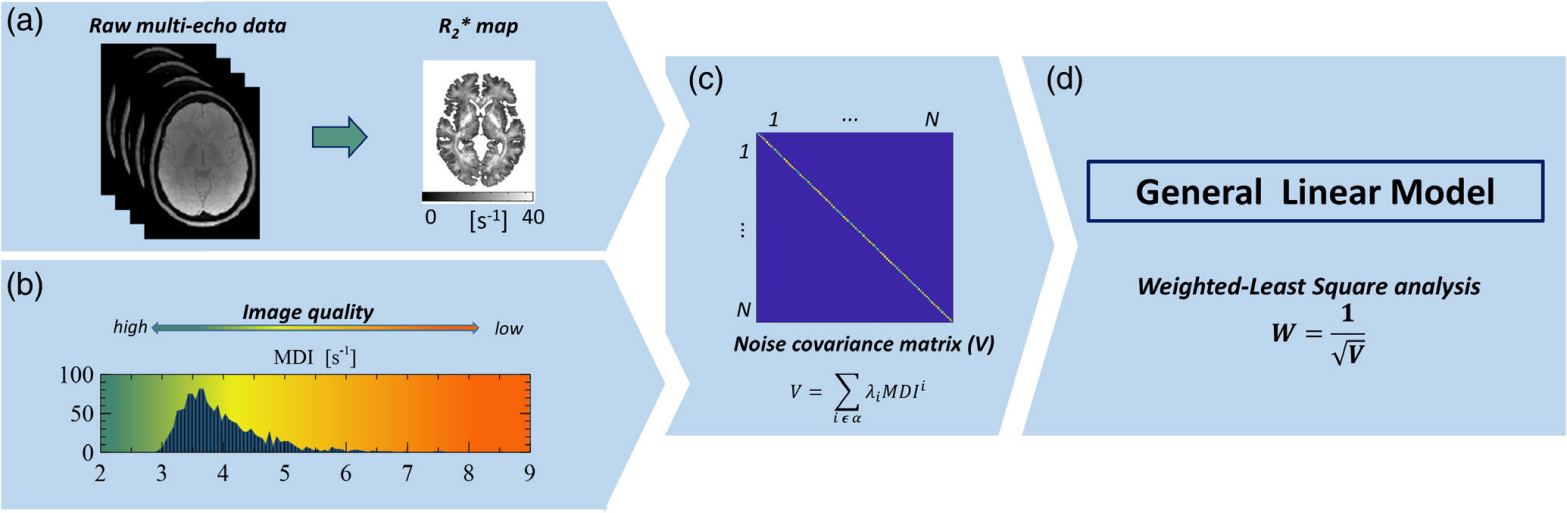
QUIQI integrates correction of motion degradation into the analysis of MRI data. For the current application, analysis data are quantitative maps of the MRI parameter R2* (a). QUIQI requires a value of the MDI for each data set of the analysis. Here, we show the distribution of the MDI values across the images used for analysis (*N* = 1,432) (b). With QUIQI, basis functions are computed from powers of the MDI and inserted into REML (Friston et al., 2002) for the computation of the noise covariance matrix *V* (c). The set of powers of the MDI, α, is pre‐defined by the user. From *V*, weights are computed that are used in the general linear model for data analysis (d)
